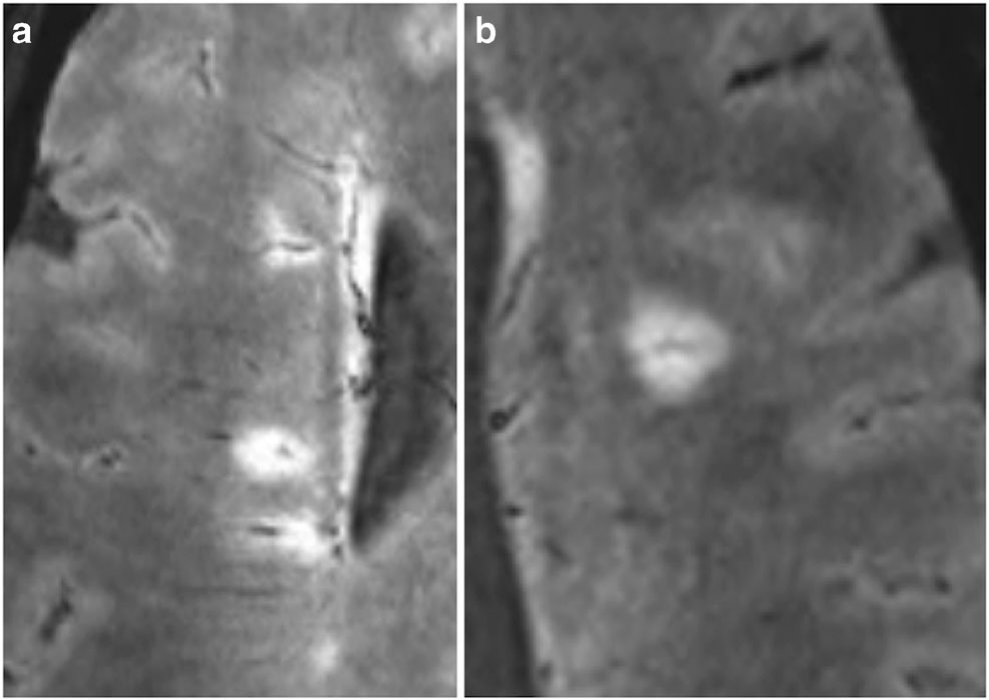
VIL^+^ lesions on FLAIR* in a patient with multiple sclerosis. (a) Multiple periventricular VIL+ lesions. (b) Subcortical VIL+ lesion. *VIL* vein in lesion
